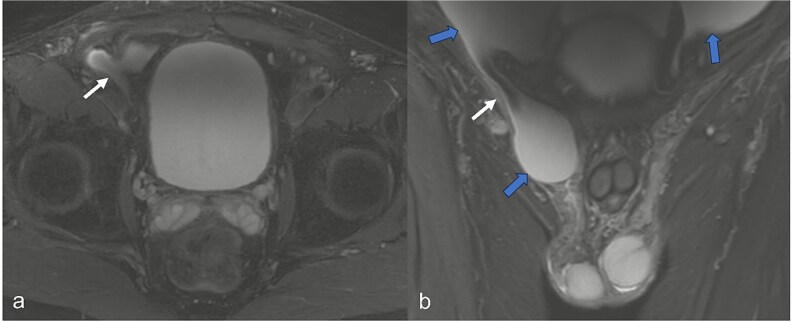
In the MRI examination of the pelvic region, the fat-suppressed, fluid-emphasized imaging in the axial section (a) shows a hernial orifice of ~1 cm (white arrow) and in the cronar section (b) the hernial orifice (white arrow) with ascites in the lesser pelvis and in the hernial sac/funiculus spermaticus (blue arrows).
